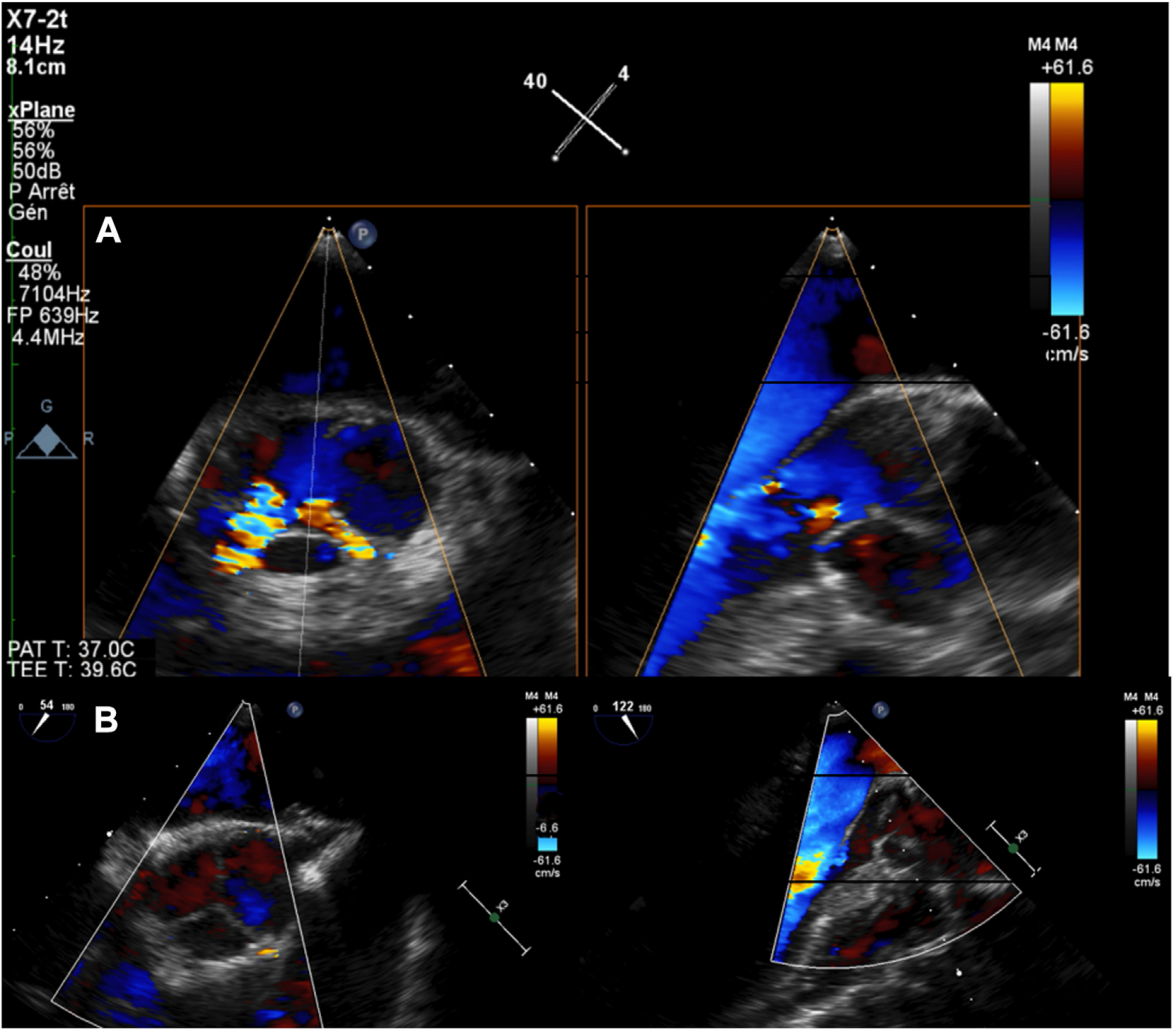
(A) Postoperative transesophageal echocardiography revealed moderate aortic insufficiency after the first aortic cross-clamping. (B) Shown is trivial aortic regurgitation after the second aortic cross-clamping.
